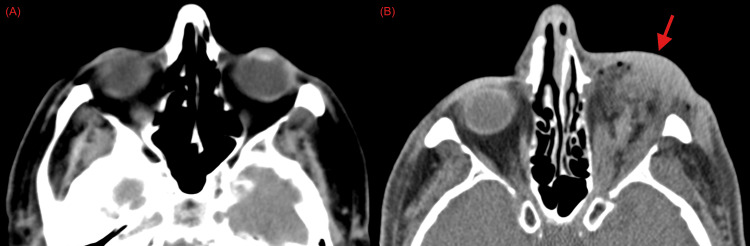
CT brain without contrast, including orbits (A) Initial imaging before self-enucleation. (B) Subsequent repeat imaging after self-enucleation with missing globe (red arrow).
